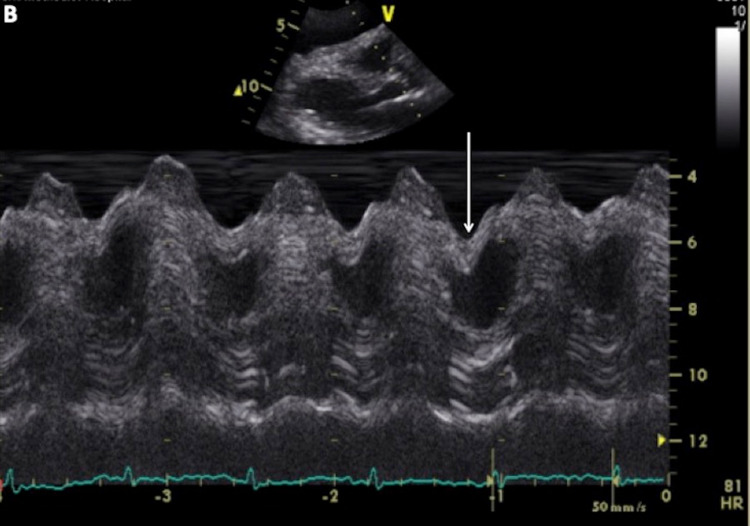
M-mode echocardiogram image in the parasternal long axis view demonstrates the right ventricle diastolic collapse (arrow)
